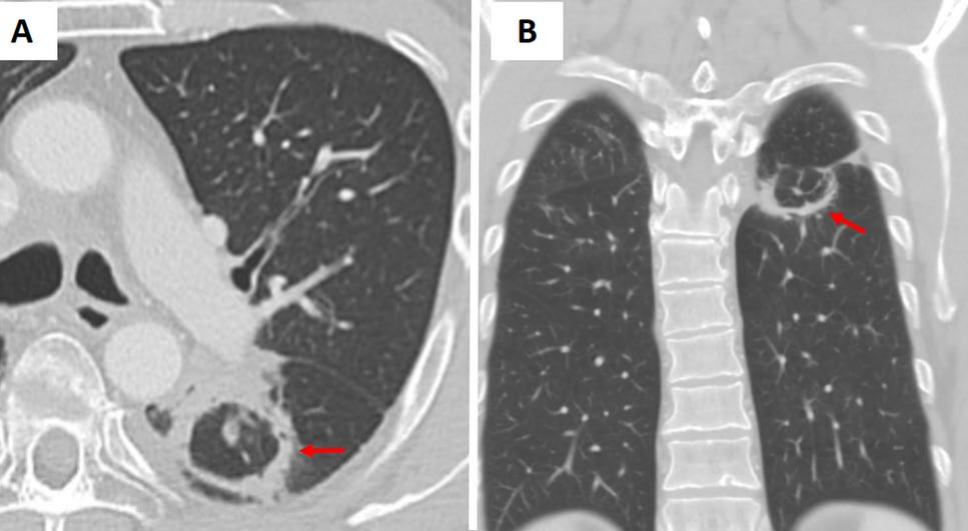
Reverse halo (atoll) sign in a patient with metastatic left lung squamous cell carcinoma who underwent hilar radiotherapy and developed radiation-induced organizing pneumonia (RIOP). A Axial and (B) coronal chest CT images demonstrate a pulmonary opacity with the atoll sign (arrows) adjacent to the irradiated left hilum
